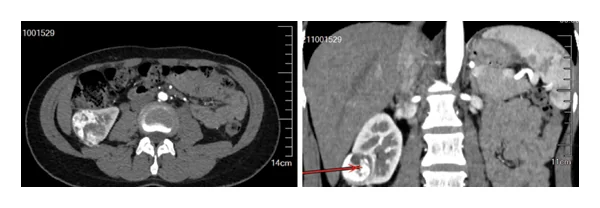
Enhanced CT features of the tumor. A slightly hypodense mass is observed at the lower pole of the right kidney. The mass exhibits heterogeneous density with indistinct margins, with a size of 42 × 30 × 33 mm, partially protrudes beyond the renal contour. The tumor demonstrates marked heterogeneous enhancement during the arterial phase on contrast‐enhanced scan. The enhancement effect diminishes during the venous and delayed phases. Cystic density is observed within the lesion.
